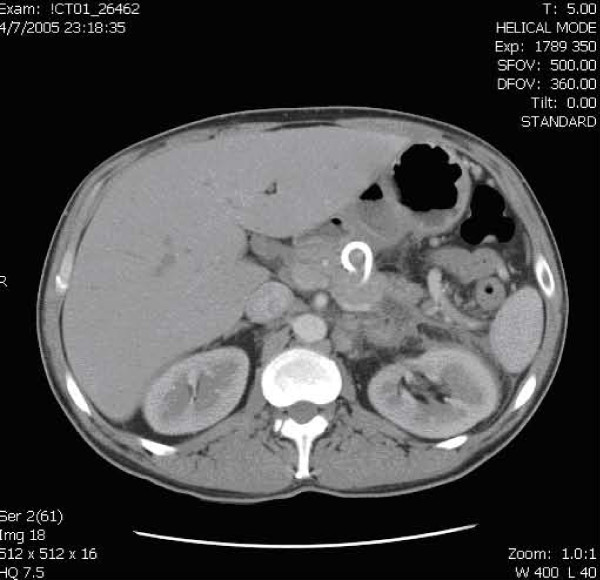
CT of the abdomen 7 months later after insertion of the transgasticstent. The pseudocyst now measures just 17 mm in size and the stent is still in place.
